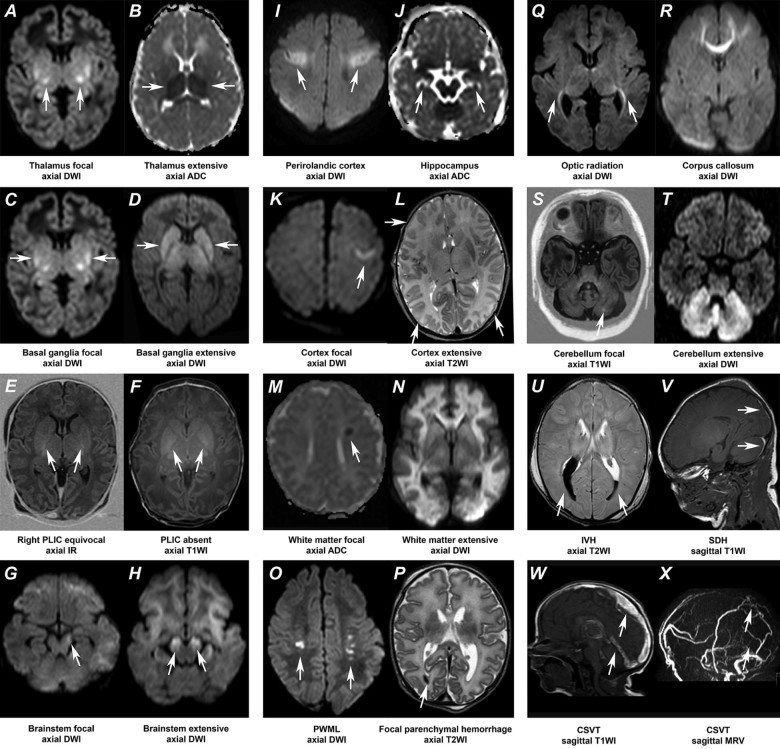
MRI examples of all items to be scored with the novel MRI score. The abnormalities of interest are marked by the *white arrows*. A, Focal bilateral thalamic lesions (high signal intensity [SI]) on an axial DWI. B, Extensive bilateral thalamic lesions (low SI) on an axial ADC map. C, Focal bilateral lesions (high SI) in the basal ganglia on an axial DWI. D, Extensive bilateral lesions (high SI) in the basal ganglia on an axial DWI. E, The posterior limb of the internal capsule (PLIC) is equivocal on both sides on an axial inversion recovery (IR) image. F, Absent PLIC bilaterally seen as an inverted signal (low SI) on an axial T1-weighted image (T1WI). G, Focal lesion (high SI) in the left cerebral peduncle on an axial DWI. H, Extensive diffusion changes (high SI) in the cerebral peduncles bilaterally on an axial DWI. I, Clear involvement (high SI) of the perirolandic gyrus bilaterally on an axial DWI. J, Bilateral involvement (low SI) of the hippocampus on an axial ADC map. K, Focal involvement (high SI) of the left cortex on an axial DWI. L, Extensive bilateral involvement of the cortex, seen as loss of the differentiation between the white matter and cortical grey matter in the occipital and frontal lobes bilaterally. M, Focal unilateral abnormal signal (low SI) in the left periventricular white matter on an axial ADC map. N, Extensive involvement of the white matter (high SI) on an axial DWI. O, Bilateral punctate white matter lesions (PWML) seen as high SI on an axial DWI. P, A small focal hemorrhage in the right occipital lobe (low SI) on an axial T2-weighted image (T2WI). Q, Bilateral involvement of the optic radiation (high SI) on an axial DWI. R, Involvement of the frontal part of the corpus callosum (high SI) on an axial DWI. S, Focal lesion (high SI) in the left cerebellar hemisphere on an axial T1WI. T, Extensive involvement of both cerebellar hemispheres (high SI) on an axial DWI. U, Bilateral intraventricular hemorrhage (IVH) seen as low SI on an axial T2WI. V, Subdural hemorrhage (SDH) supra- and infratentorial seen as high SI on a sagittal T1WI. W, Cerebral sinovenous thrombosis (CSVT) seen as high SI at the location of the superior sagittal and straight sinus on a sagittal T1WI. X, With corresponding lack flow (lack of high SI) in those veins on an MR venography (MRV) in sagittal view.
